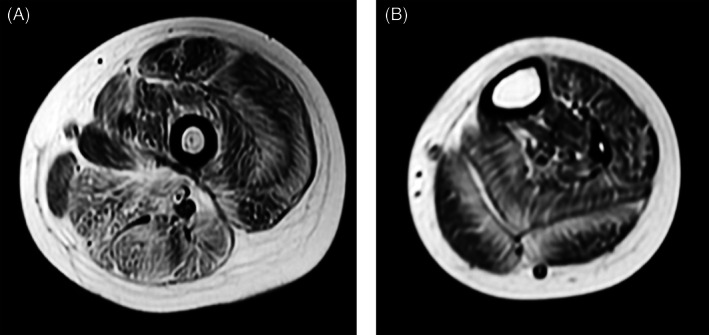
(A, B) High intensity observed on T1‐weighted magnetic resonance imaging in the peripheral area of the vastus lateralis (white arrow), soleus, and gastrocnemius muscles (white arrow head), in addition to the central area of the rectus femoris muscle (black arrow head)
